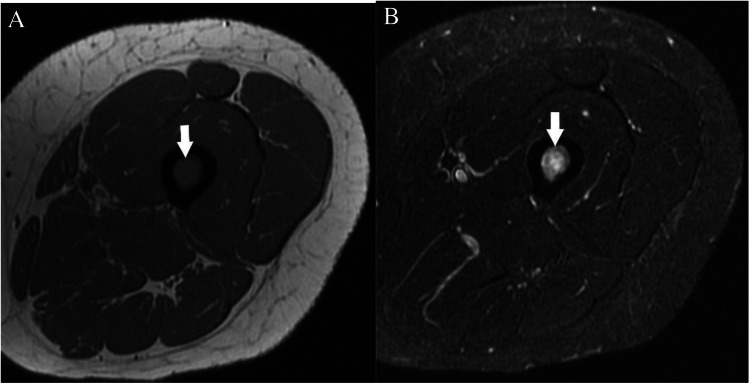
Case 3. Breast cancer metastasis. 57-year-old female runner presents with thigh pain. There is a lesion (arrows) in the midshaft of the femur that is isointense to skeletal muscle on the A axial T1-weighted MR image and hyperintense on the B axial T2-weighted fat-suppressed MR image
